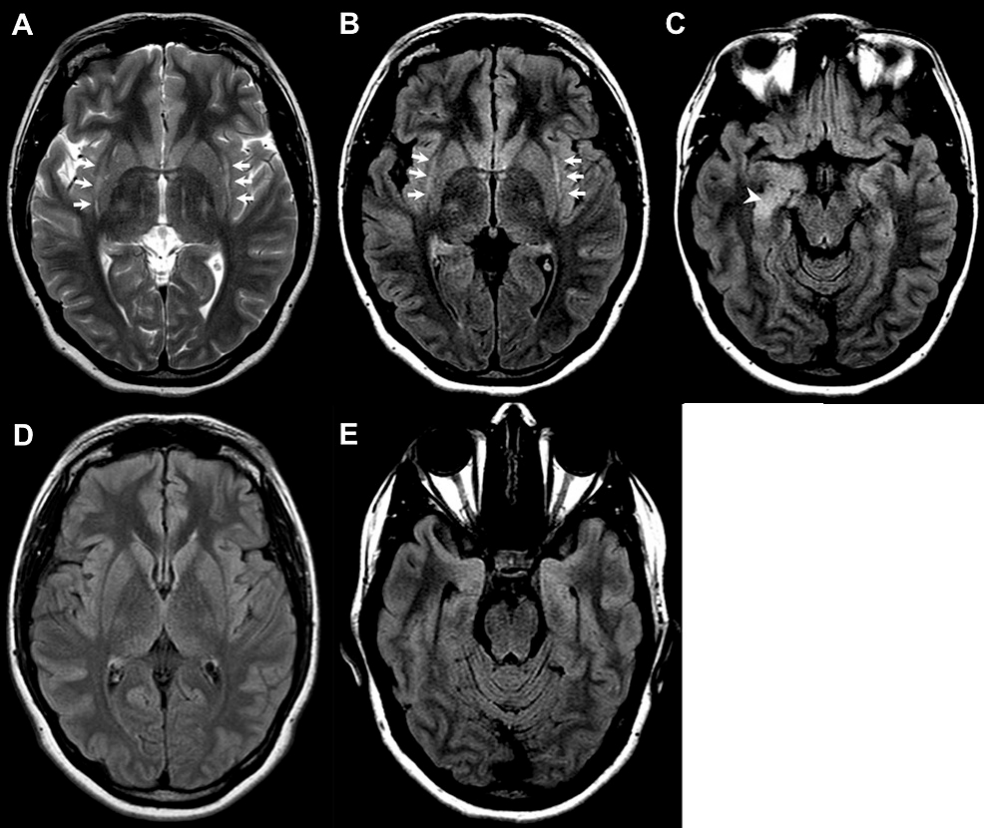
Axial T2-weighted and FLAIR images at presentation and three months later Axial T2-weighted (A) and FLAIR (B, C) images demonstrate bilateral claustral hyperintensities (arrows), and asymmetric hyperintensity of the right mesial temporal lobe (arrowhead). FLAIR images three months later (D, E) showed normalization of claustral and mesial temporal lobe signals. FLAIR: fluid-attenuated inversion recovery.
